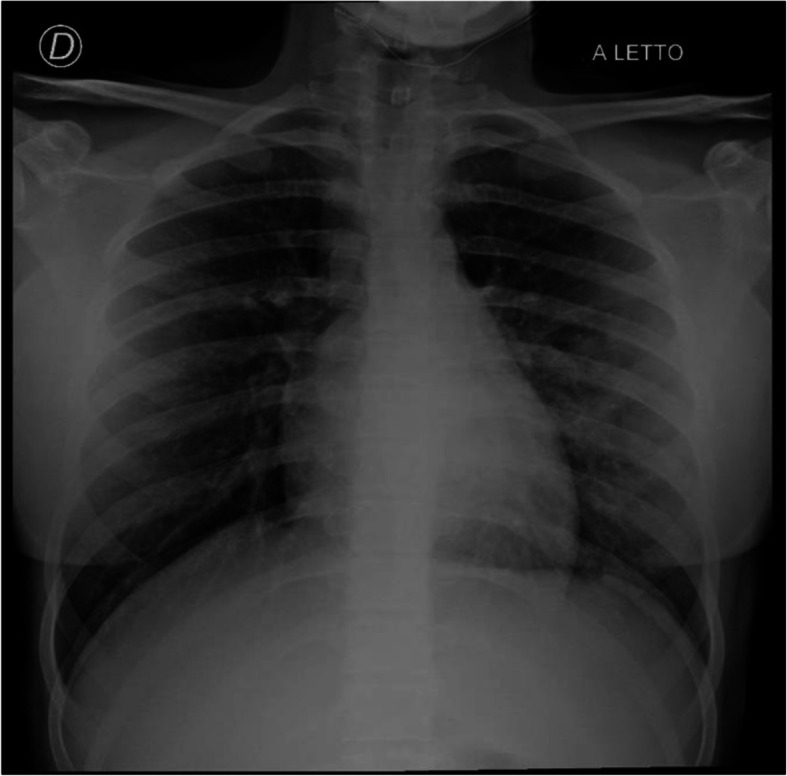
Patient’s chest X-ray: Interstitial involvement marked in hilar and basal left regions
